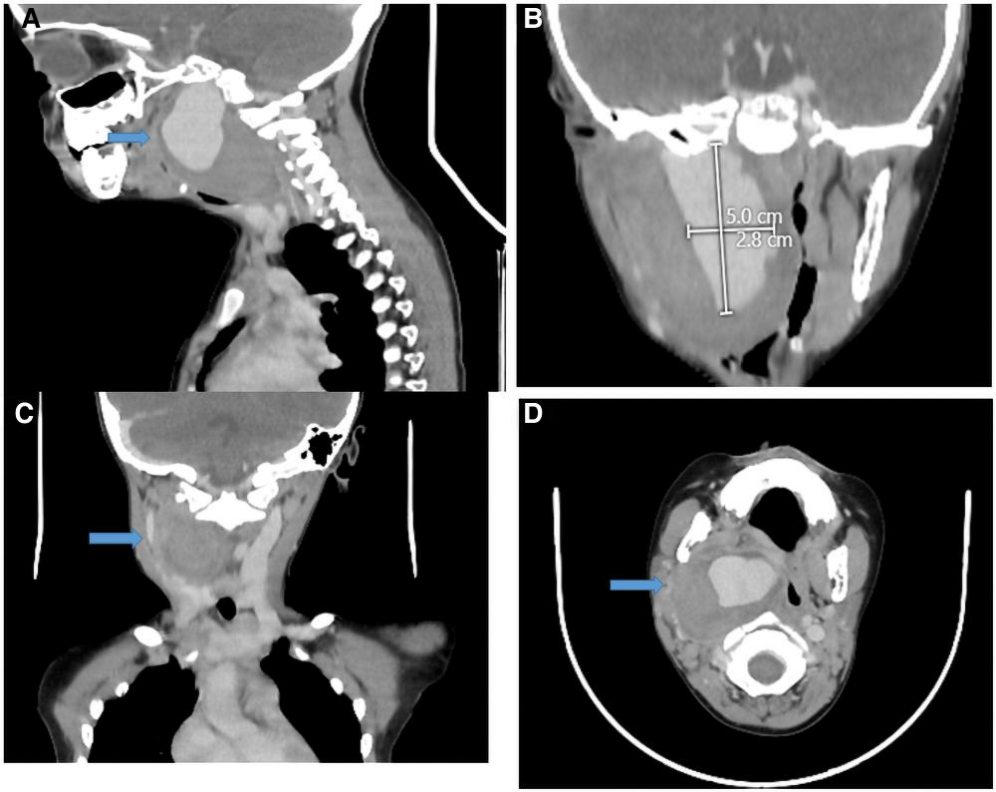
Contrast-enhanced CT scan of the neck demonstrating a pseudoaneurysm of the right internal carotid artery with surrounding parapharyngeal haematoma.
